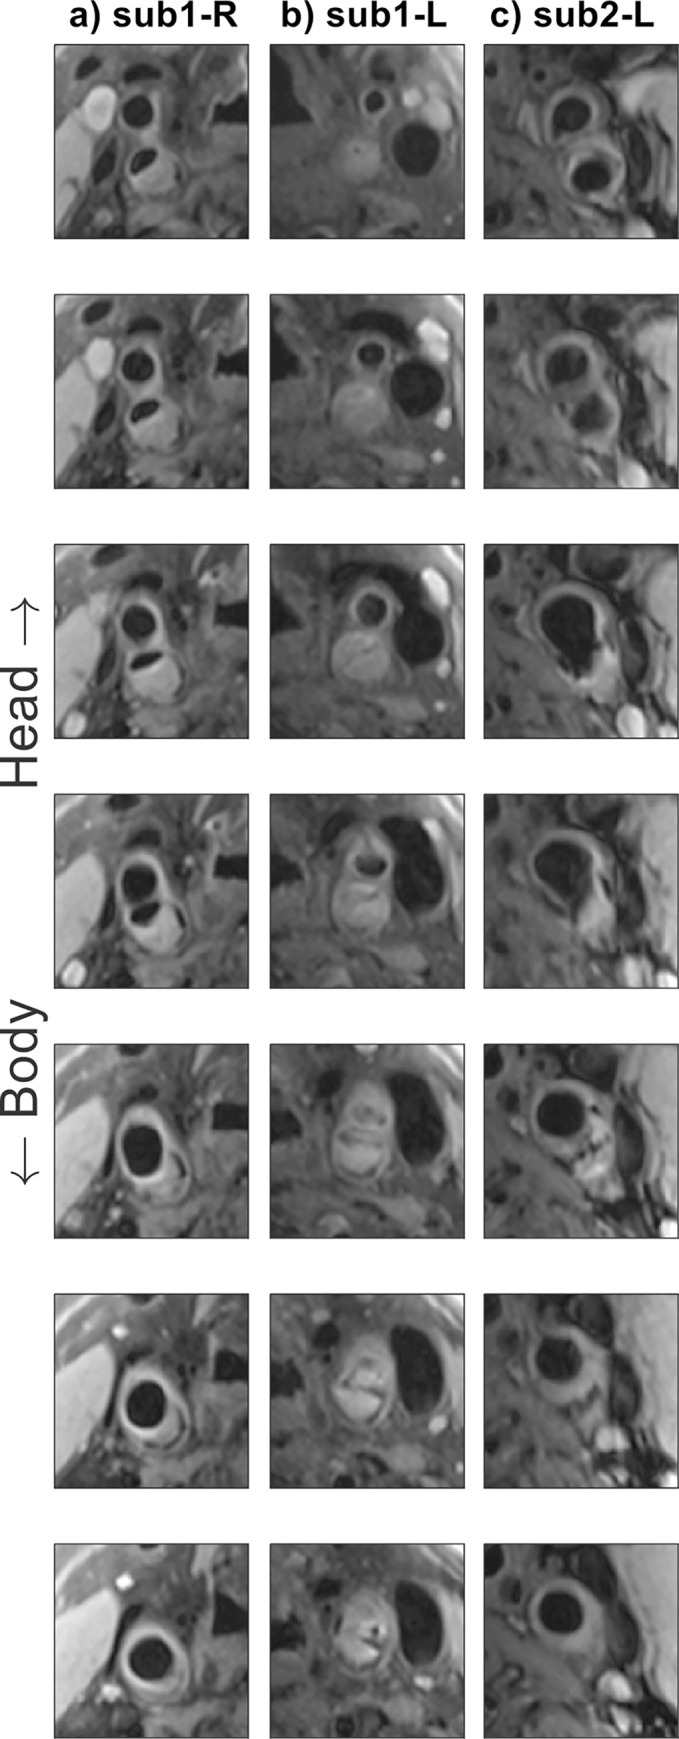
Consecutive DANTE-FSE T1-weighted slice segments showing the carotid bifurcations in two patients with atherosclerotic carotid artery disease, acquired using the new 10-channel coil. (a-b) Subject 1, left- and right-hand sides; (c) subject 2, right-hand side.
